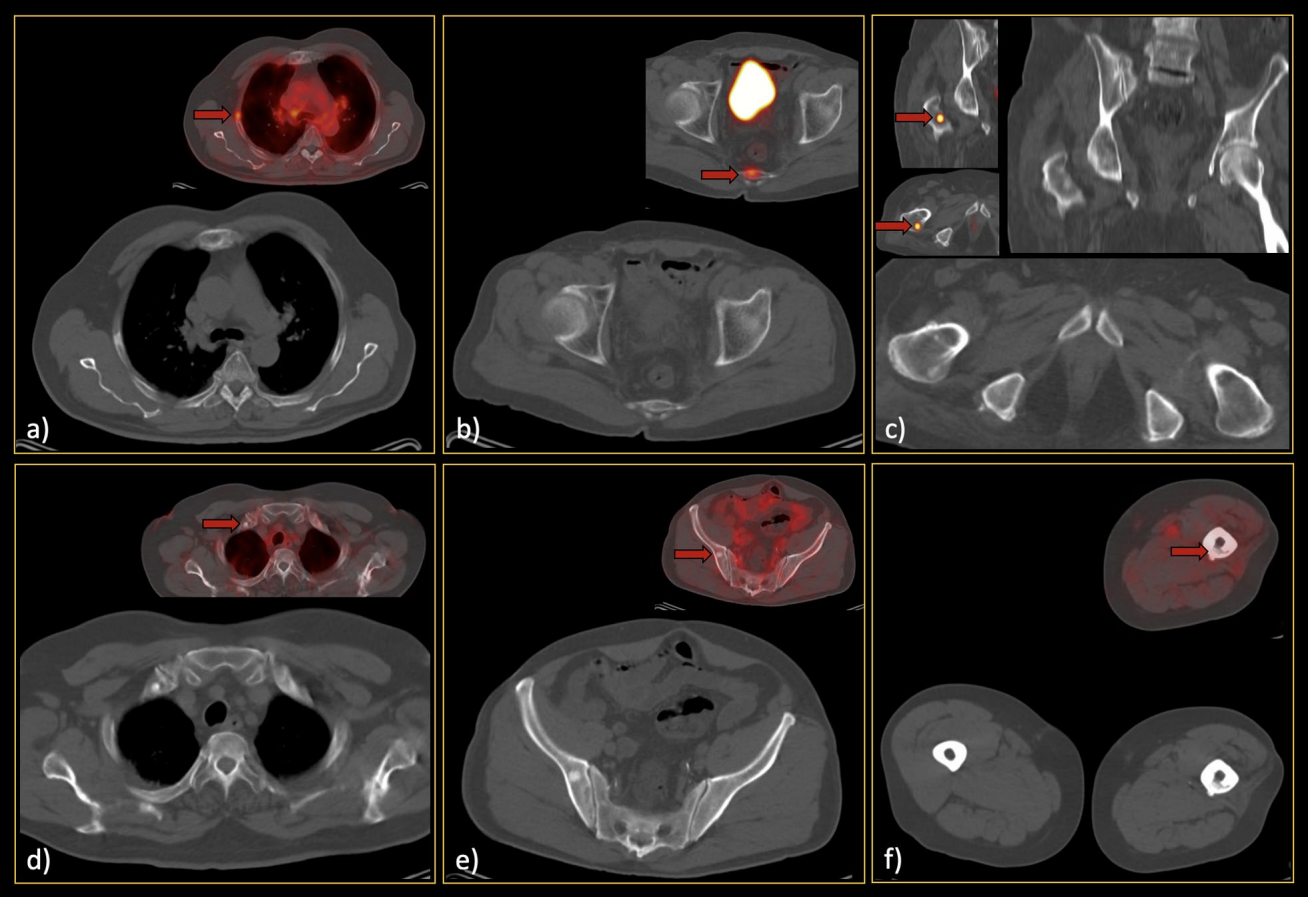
Lesions that were wrongly classified (a–c false negatives; d–f false positives) by one of the readers while correctly diagnosed by others. Thus, attention to these locations/kinds of lesions should be paid since they may also be missed on CT readings. Red arrows show the location of the metastases on the hybrid ^68^Ga-PSMA PET/CT images
